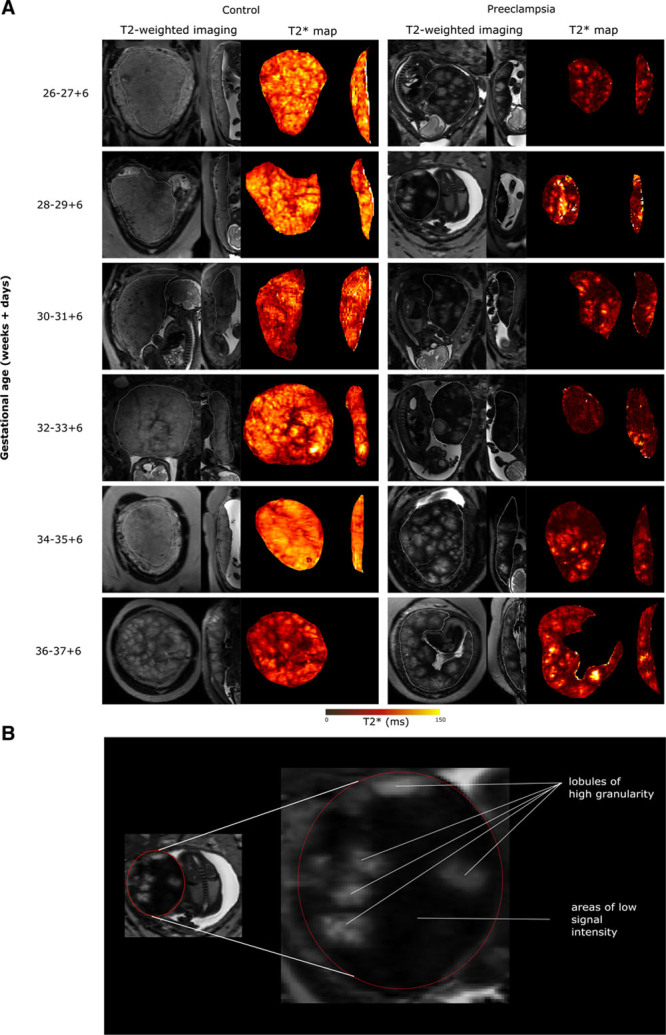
T2-weighted imaging and T2* maps. A, Example T2-weighted imaging and T2* maps in coronal and sagittal planes across gestation. B, Features of T2-weighted imaging in women with preeclampsia.
